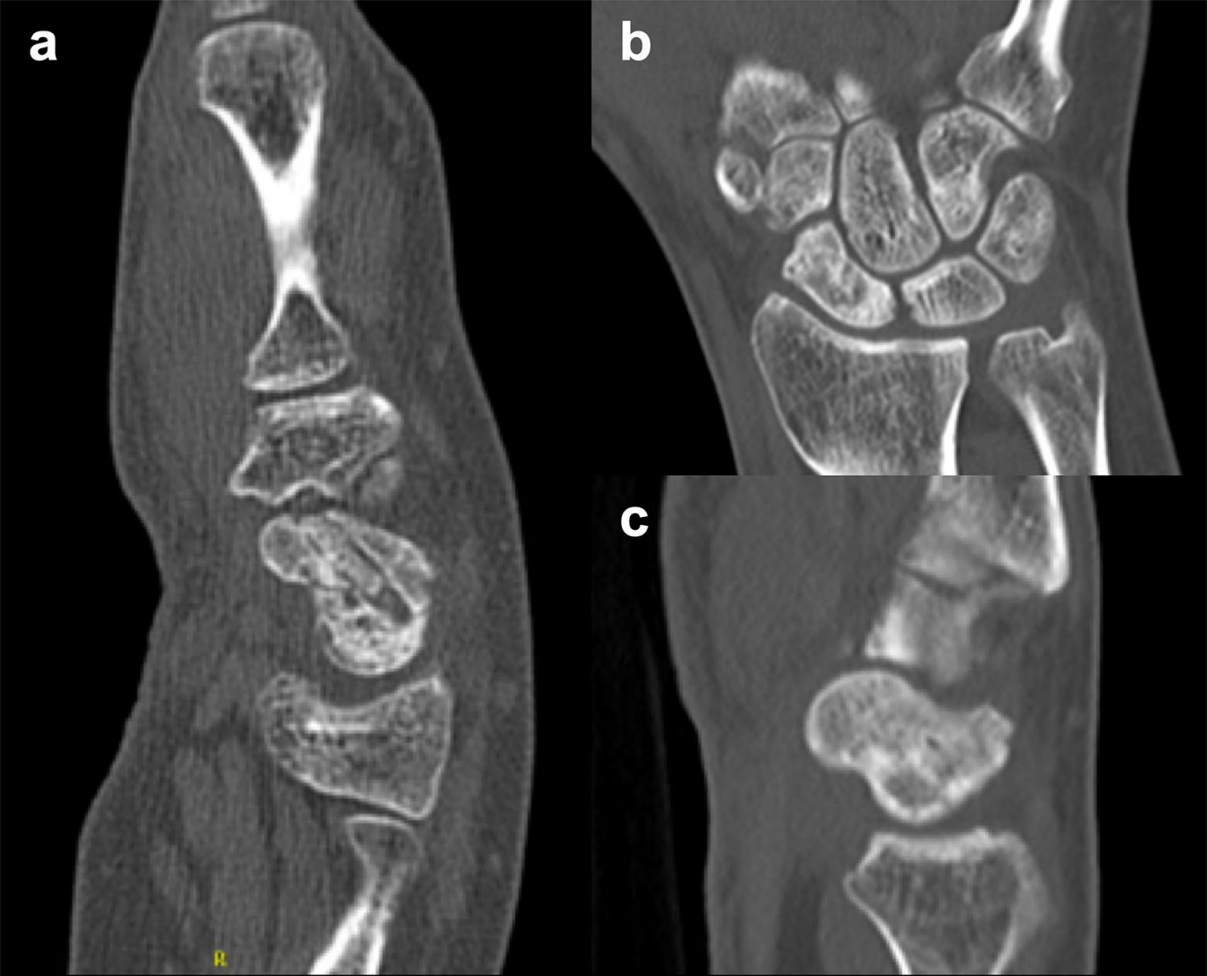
Postoperative CT scans of patient #2. a Sagittal view 12 weeks postoperatively with dowel along the scaphoid axis and beginning consolidation. b coronal and c. sagittal view 11 months postoperatively with good integration of the dowel to the consolidated scaphoid
